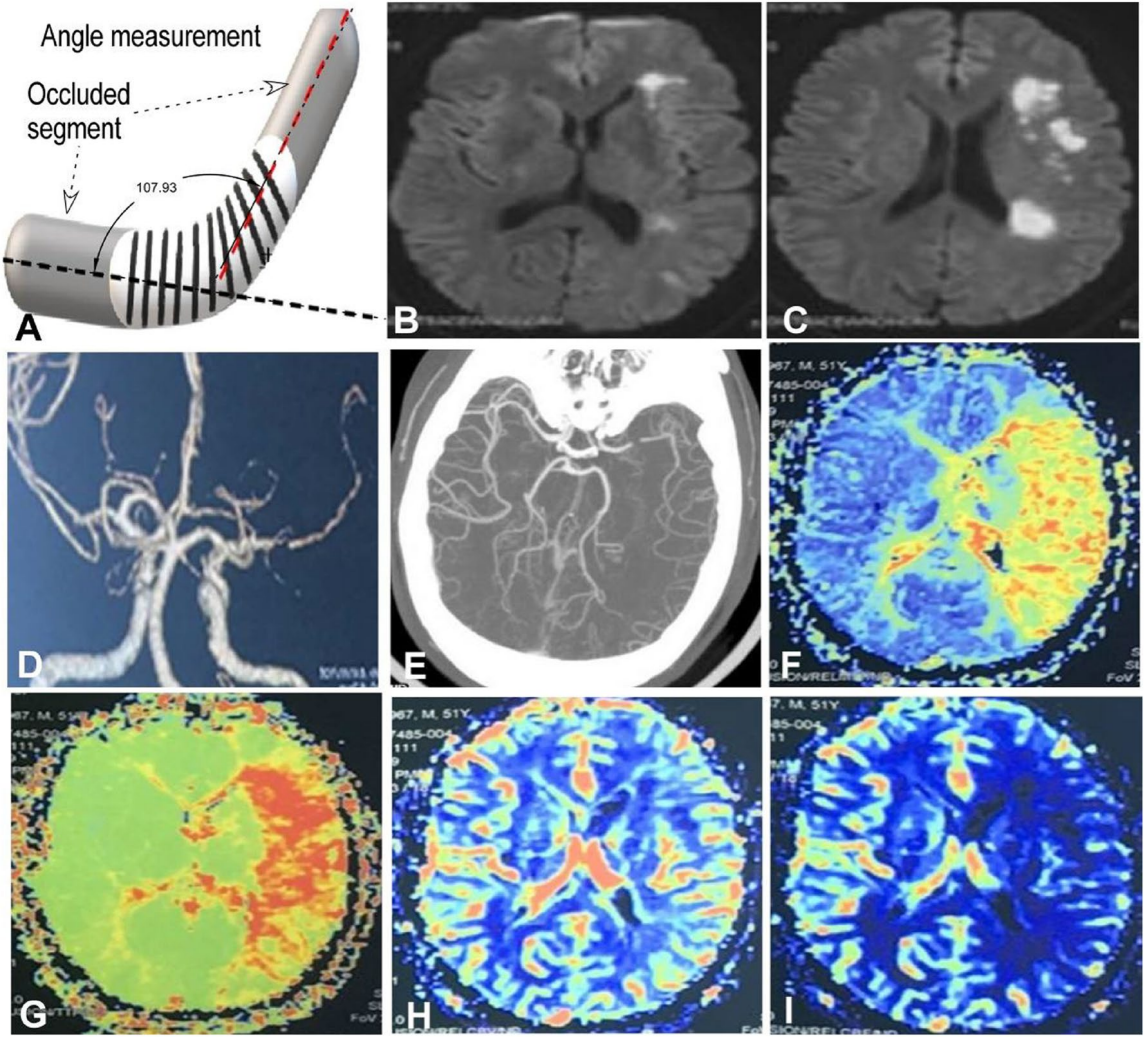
Measurement of angulation at the occluded arterial segment and case presentation. (A) The angle formed betwwen the extending line of the proximal segment and that of the distal segment measured 107.93°. (B–I) A male patient aged 51 years with hypertension for 6 years complained inability to walk, right limb weakness, and unclear speech for 2 days. Physical examination showed the muscle strength of the right limb of grade 2 and normal muscle tension. The modified Rankin scale (mRS) score was 4. (B,C) Preoperative magnetic resonance imaging (MRI) showed acute cerebral infarction in the left basal ganglia and semioval center. D&E. Cerebral computed tomographic angiography (CTA) revealed occlusion of the M1 segment of left middle cerebral artery. (F–I) Head MRI perfusion weighted imaging (PWI) confirmed low perfusion in the blood supply area of the left middle cerebral artery. (F) Mmean transit time; (G) time to peak; (H) cerebral blood volume; (I) cerebral blood flow.
